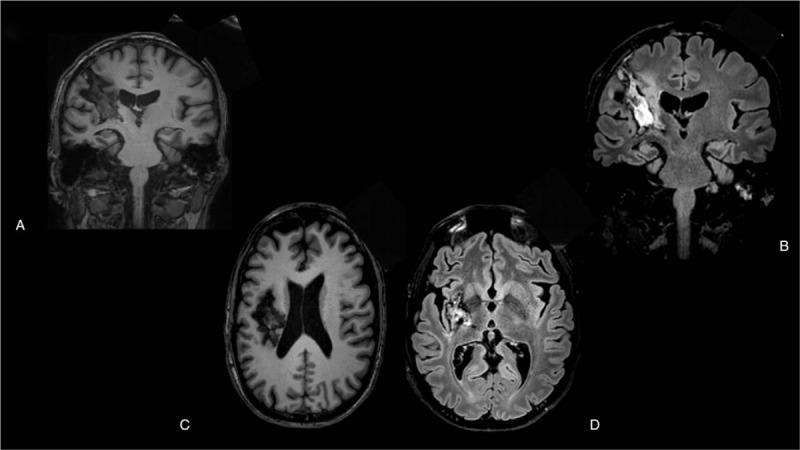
(A) Coronal T1-weighted image. (B) Coronal fluid attenuated inversion recovery (FLAIR)-weighted image. (C) Axial T1-weighted image. (D) Axial FLAIR-weighted image.
